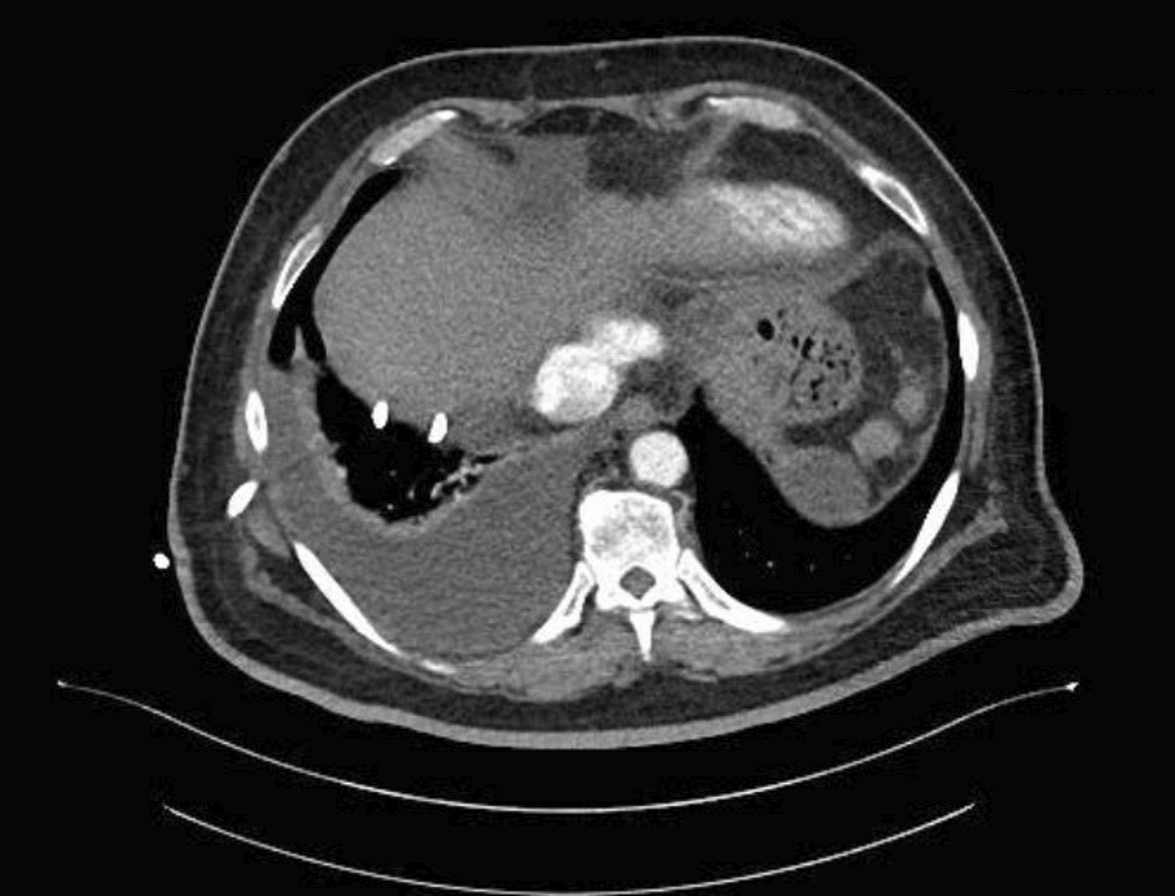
Computed tomography (CT) of the thorax demonstrating right pleural effusion with chest drain in situ. No lung mass or pleural nodularities were noted.
